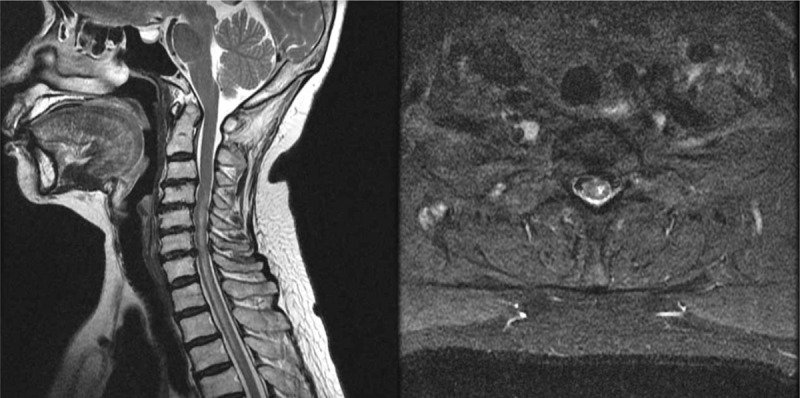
(A) Sagittal T2-weighted image of the cervical spine, intramedullary high signal intensity is seen at left sided spinal cord from C4 to T4 with ill-defined edema. (B) axial T2-weighted image of the cervical spine, showing intramedullary high signal intensity.
